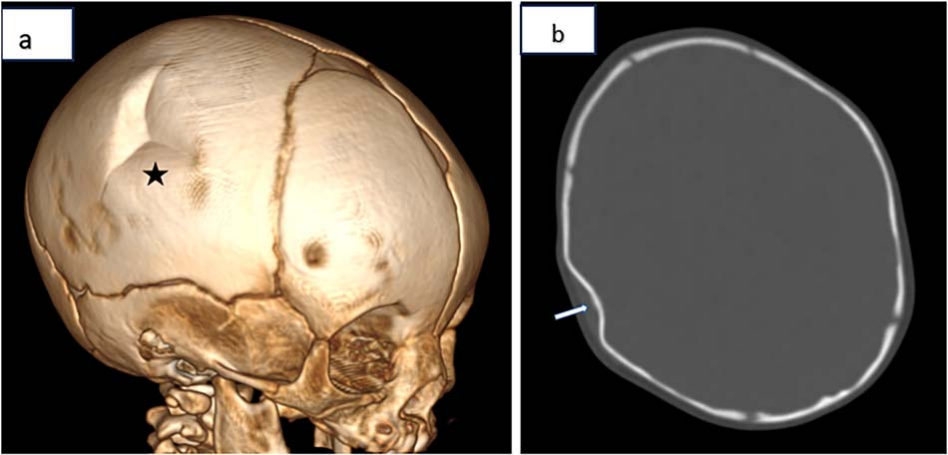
(a and b) Three-dimensional volume-rendered reconstruction and Axial CT showing the typical appearance of a ping-pong ball fracture (Star) and a depression of the right parietal bone consistent with a ping-pong ball fracture (Arrow).
